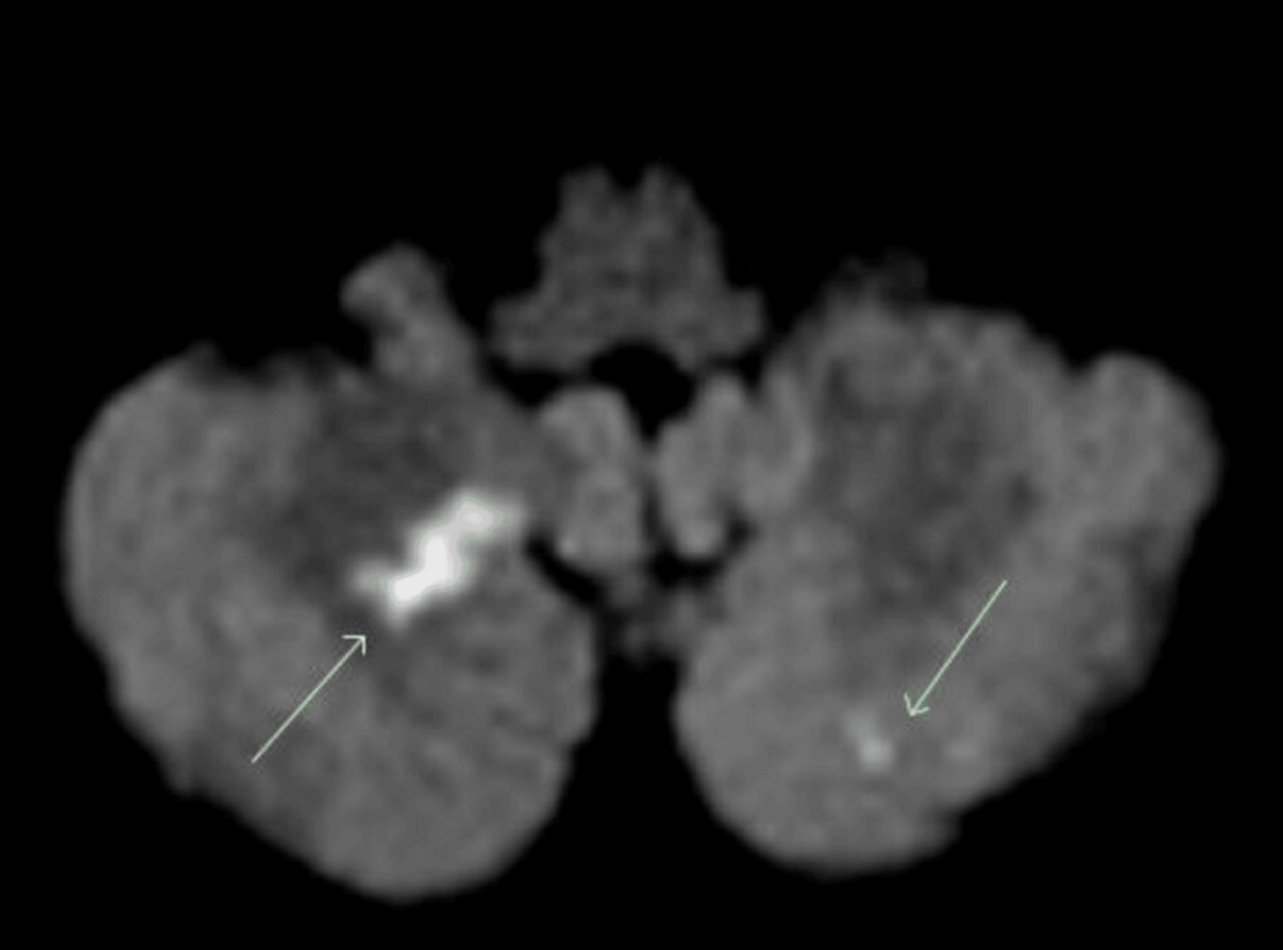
Axial magnetic resonance imaging of the brain demonstrating multiple punctate areas of restricted diffusion consistent with acute infarction in the bilateral cerebellar hemispheres.
